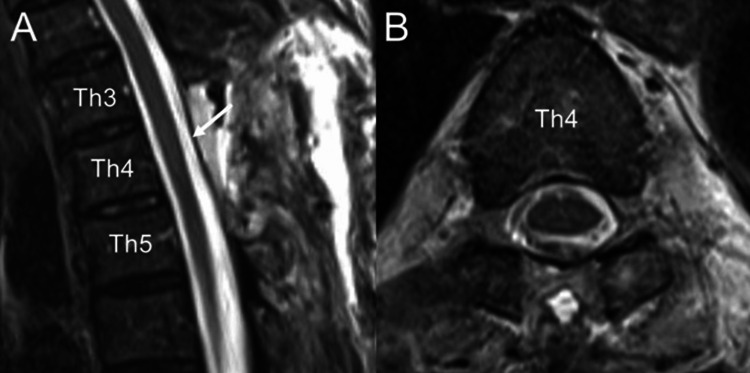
(A, B) Sagittal and axial T2-weighted images show the realignment of the spinal cord following the resection of the SAW (white arrow). SAW: spinal arachnoid web
